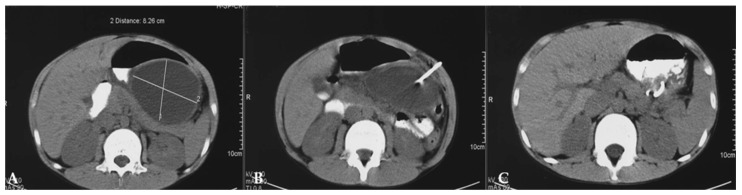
External drainage of huge pancreatic pseudocyst: (A) Pseudocyst prior to drainage (8.3 × 6.1 cm); (B) CT-assisted extranal percutaneous drainage; (C) Complete resolution of the cyst after six weeks.
